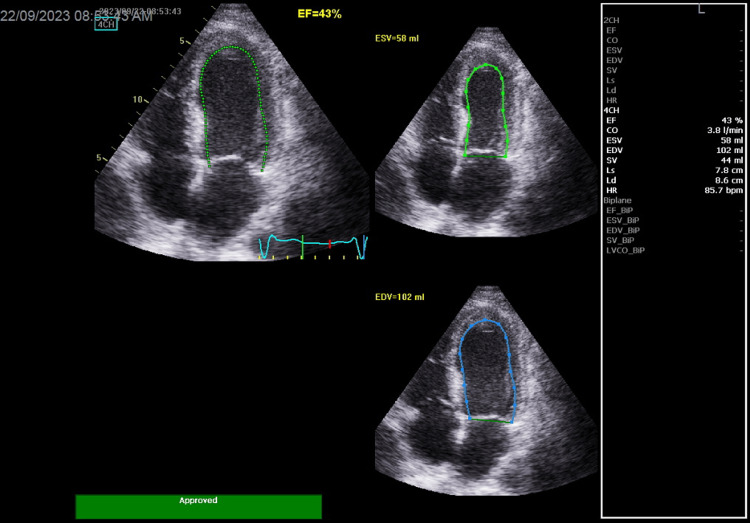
Echocardiogram done post MI and four days post PCI showing akinesia of the septum, apex, and mid-anterior segment with an LVEF at 43%. No left atrial and no left ventricular thrombi were noted. MI: myocardial infarction; PCI: percutaneous coronary intervention; LVEF: left ventricular ejection fraction.
